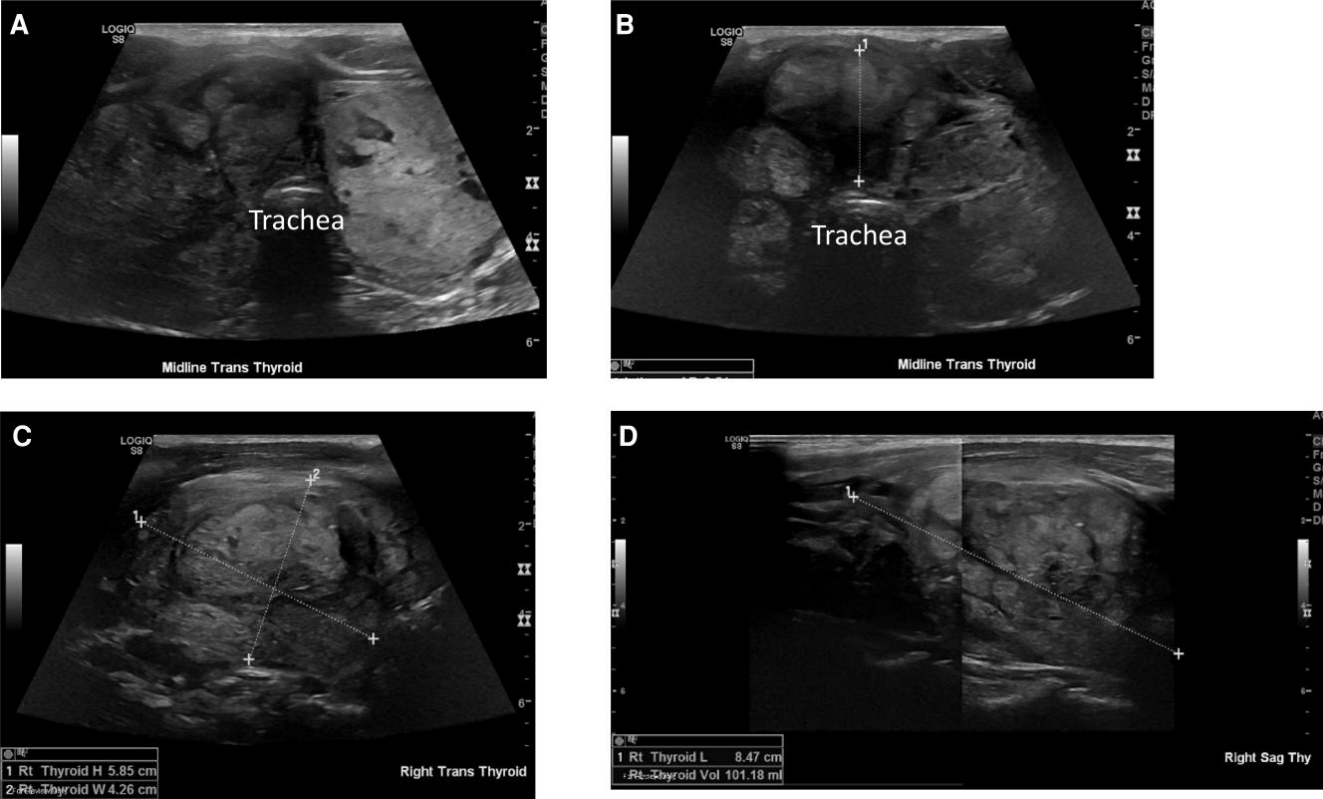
Thyroid ultrasound images 8 weeks after radiofrequency ablation of right and isthmic nodules. (A) Thyroid in transverse plane; (B) isthmus nodule; (C) right nodule in transverse and sagittal (D) views.
